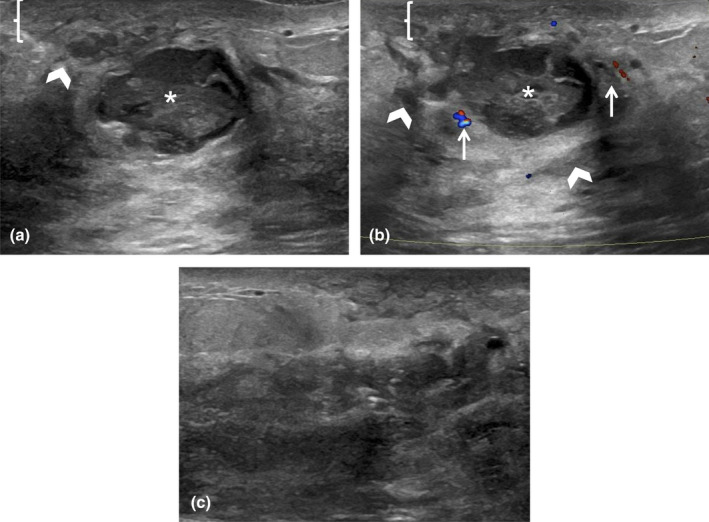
25‐year‐old lactating woman initially felt a 25 mm firm lump in her right breast, which progressively increased in size over 1 week. Ultrasound was performed with an ML6‐15 MHz transducer with the frequency set to 11 MHz, and the images were acquired from the skin to the chest wall. Targeted ultrasound of the redness in the subareolar right breast showed mild skin thickening measuring 4 mm (bracket), oedematous hyperechoic fibroglandular tissue (chevrons) and a discrete complex fluid collection measuring 4.4 cm (asterisk) (a and b). There was also mild surrounding increased vascularity (arrows) (b). Findings were consistent with an abscess, which was confirmed on subsequent percutaneous ultrasound‐guided aspiration, with the near‐complete collapse of the abscess cavity post‐aspiration (c). 15 mL of purulent fluid was aspirated with an 18‐gauge needle and sent to the laboratory to obtain organism sensitivities prior to the initiation of antimicrobial therapy. The patient's symptoms continued and required 2 additional aspirations at 3 and 6 days later.
